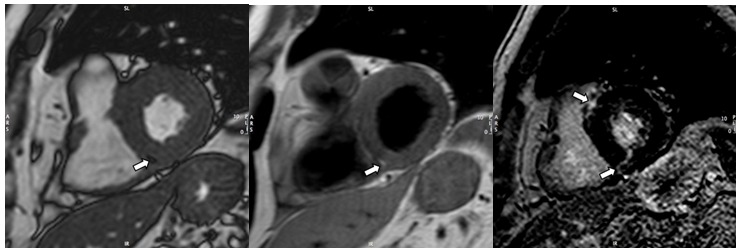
Cardiac MRI. *On the left*. Short-axis cine image: focal intramyocardial area showing “indian-ink sign” (arrow). *At the Center*. Fast-spin echo T1-weighted image obtained at the same level: area of signal hyperintesity (arrow) compatible with fatty infiltration. *Right*. Late post-gadolinium short-axis image with focal intramyocardial areas of hyperenhancement (arrows) involving the septal interventricular junctions (suggestive for myocardial fibrosis with non-ischemic pattern of distribution).
